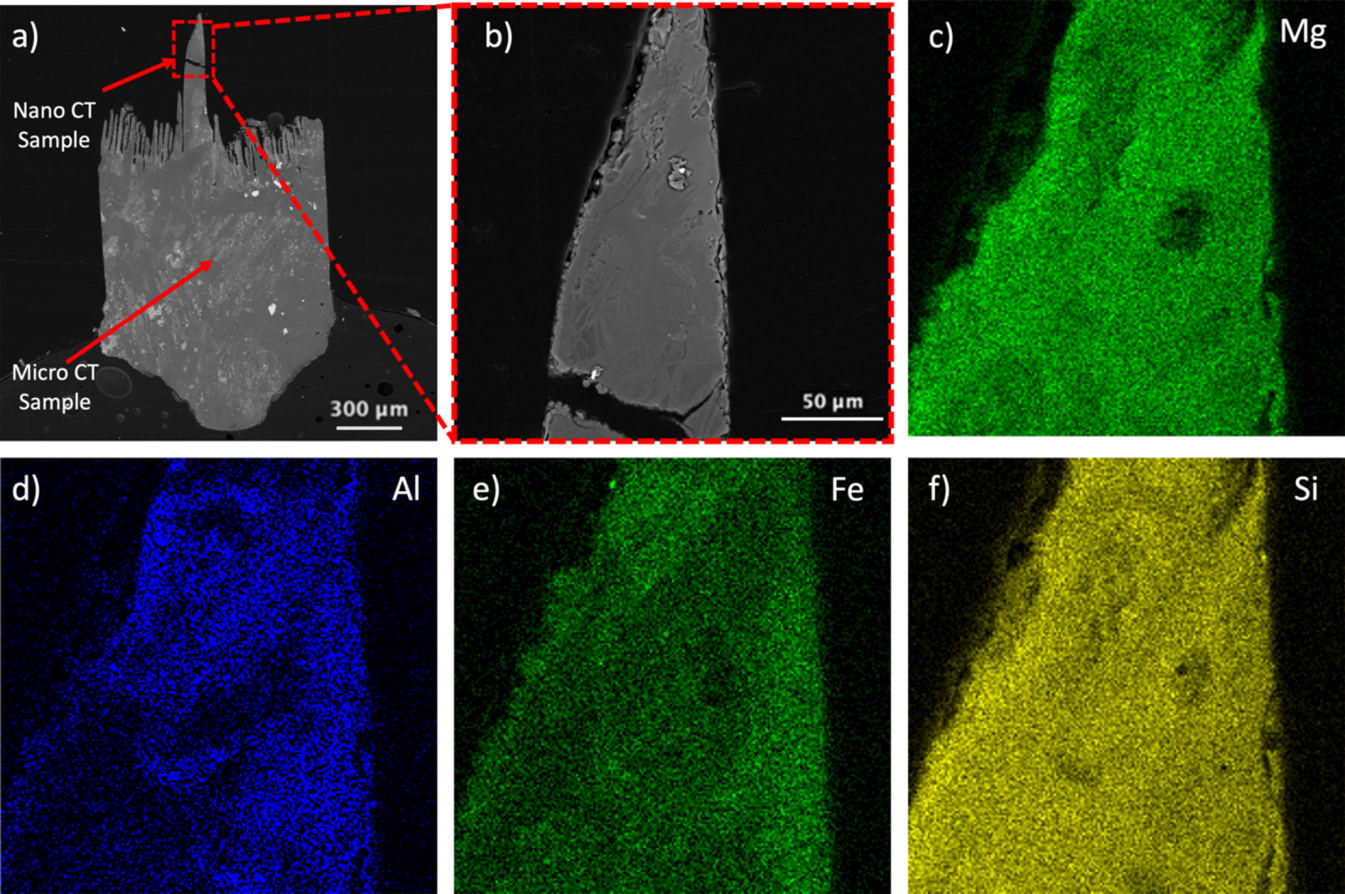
SEM BSE image of (*a*) the micro- and nano-CT sample and (*b*) the nano-CT sample region. Also, EDS composition maps of the nano-CT sample region for (*c*) Mg, (*d*) Al, (*e*) Fe and (*f*) Si.
